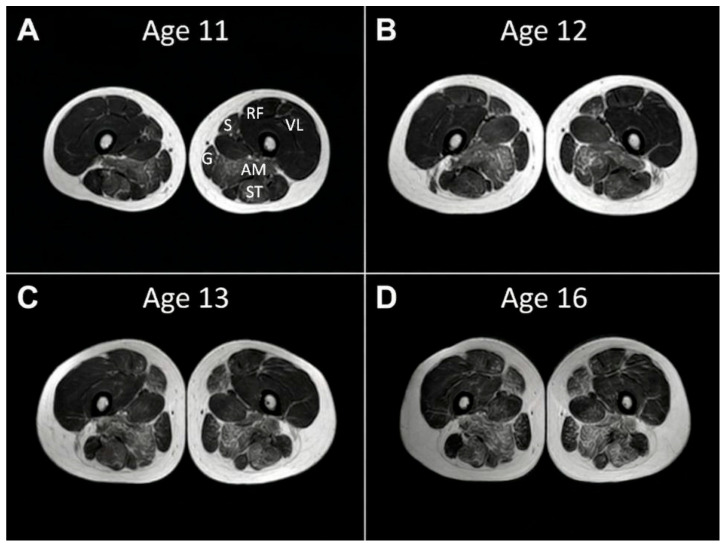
Longitudinal axial T2-weighted MRI of the thighs from age 11 to 16 years. Serial imaging demonstrates a progressive, symmetric fatty replacement with a distinctive “posterior-to-anterior gradient”. (A) Age 11: Early fatty infiltration (mild T2 hyperintensity) is confined to the posterior compartment, specifically the adductor magnus (AM) and semitendinosus (ST). (B) Age 12: Dystrophic changes progress in the posterior compartment, while the rectus femoris (RF), vastus lateralis (VL), sartorius (S), and gracilis (G) remain preserved. (C) Age 13: Accelerated fatty replacement is noted in the AM and hamstrings. Notably, the S and G begin to exhibit hyperintense streaking, marking the onset of involvement. (D) Age 16: Complete fatty replacement (Mercuri grade 4) involves the entire medial and posterior compartments (AM, ST, S, and G). In stark contrast, the anterior compartment (RF and VL) remains remarkably intact with preserved muscle volume and normal low T2 signal intensity.
